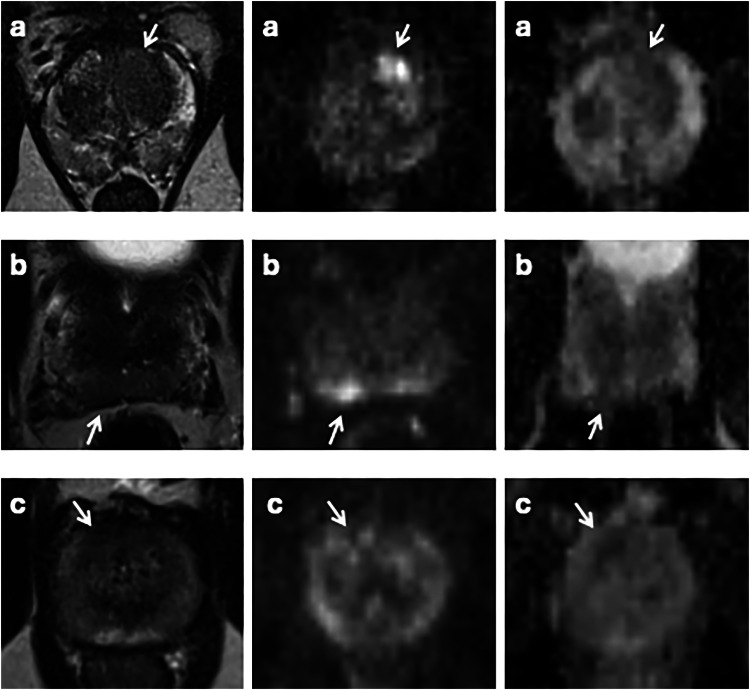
Three cases (a–c) of GG ≥ 2 PCa detected by the bpPI-RADS ≥ 4 pathway but missed by both the ‘stage-gated’ reading and Likert ≥ 4 pathways. The GG ≥ 2 lesion (arrow) is shown on axial T2WI (left), high *b*-value (b1500) DWI (middle), and ADC maps (right). Scans were all performed at 3-T. a A 63-year-old man with PSA 1.7 ng/mL and PSA density (PSAd) 0.05 ng/mL^2^. b A 58-year-old man with PSA 1.0 ng/mL and PSAd 0.03 ng/mL^2^. Both (a, b) were positive by only one radiologist in Stage 1. Due to normal PSAd, Stage 2 review was not performed. These cases were classified as radiologist interpretation errors. c A 59-year-old man with PSA 1.8 ng/mL and PSAd 0.05 ng/mL^2^. The screening images were negative by all radiologists in Stage 1, so they did not have Stage 2 review. In retrospect, the lesion is mainly conspicuous on the ADC map. This case was classified as a limitation of the reading strategy
